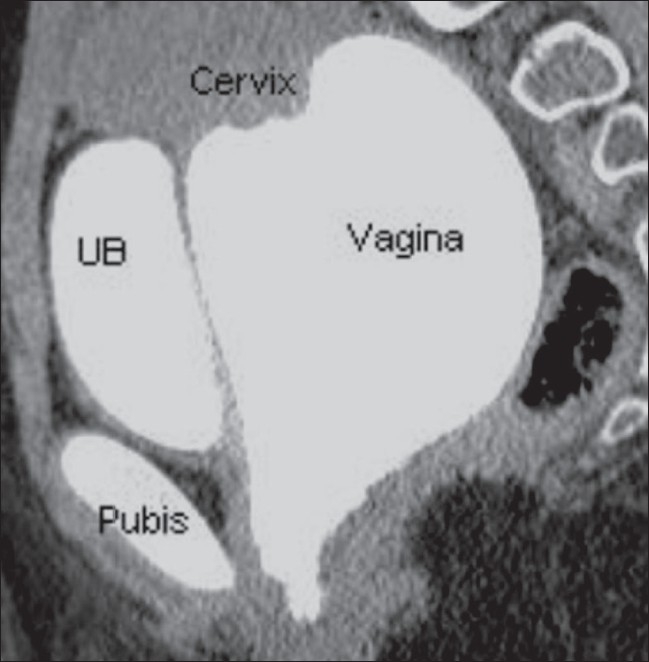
Sagittal reformatted CT scan of the pelvis shows a contrastfilled vagina, separate and posterior to the urinary bladder, without any anomalous connection/extravasation
